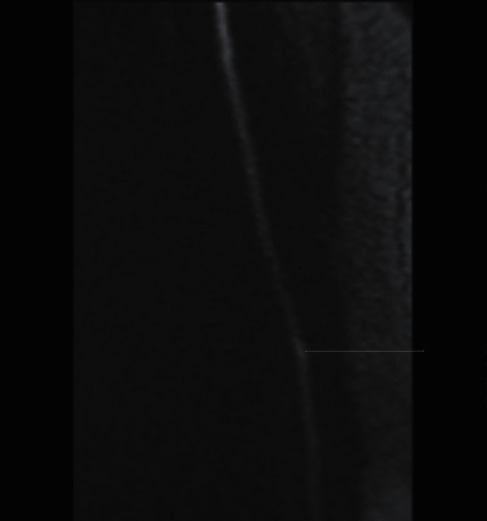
MRI diffusion-weighted imaging.
